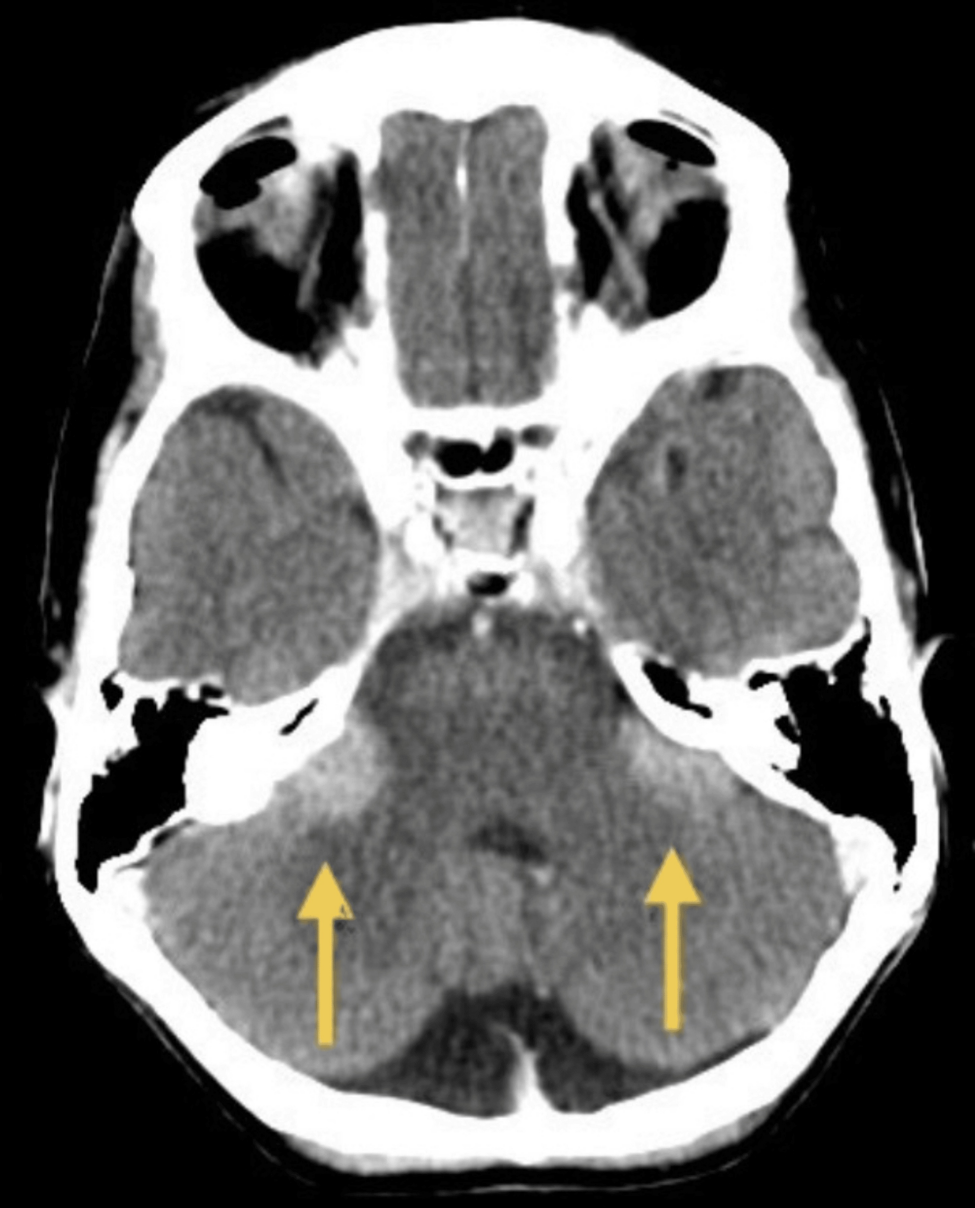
Non-contrast axial CT scan showing a cerebellopontine angle mass on the both sides. This non-contrast CT scan demonstrates a soft tissue mass involving both cerebellopontine angles. The lesion was initially overlooked on the first radiology report and was later identified following multidisciplinary review.
